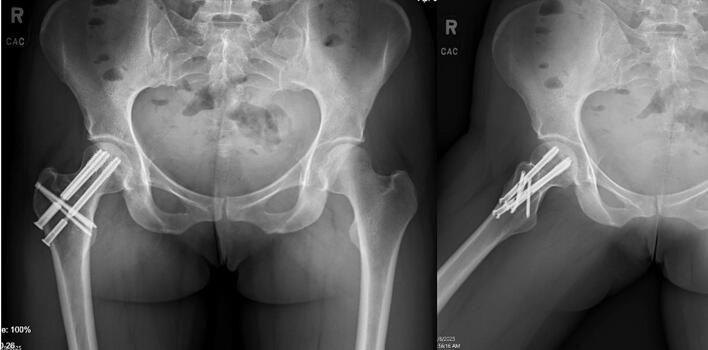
Postoperative AP and lateral radiographs from the patient's 1-year follow-up appointment demonstrating a successful radiographic outcome. The fracture has healed anatomically and there is no evidence of screw migration, cutout, or loosening.
